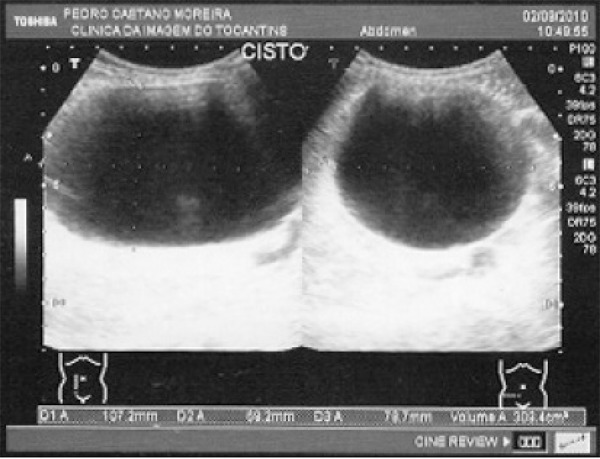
Ultrasound image of the abdomen with intraperitoneal cyst
